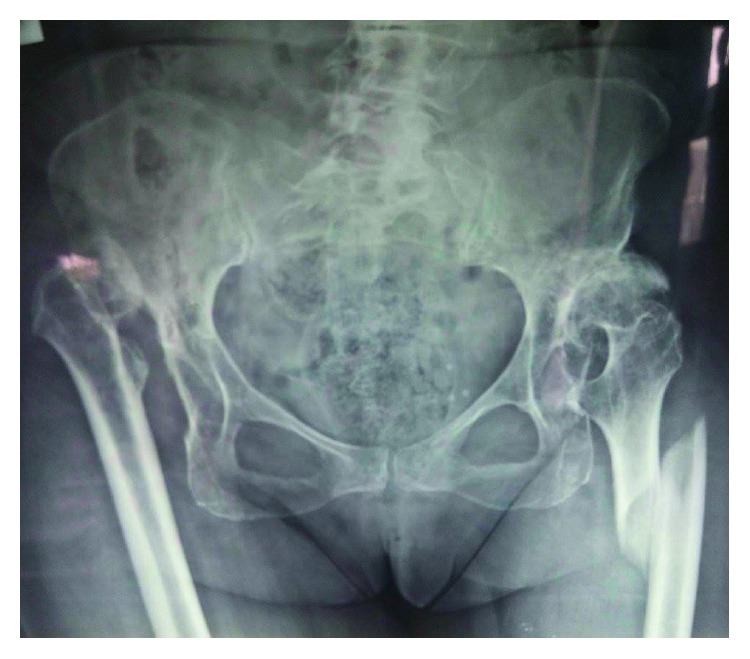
Pelvis anteroposterior radiograph showing the neglected hip dislocation of both hips.
